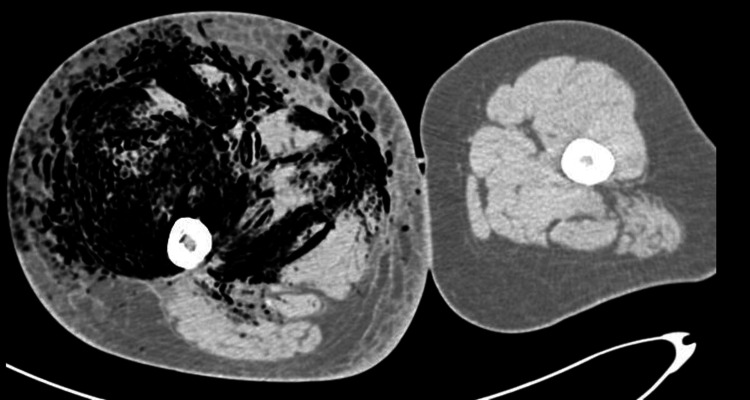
Axial CT scan of the right thigh showing severe soft tissue edema and extensive gas collections dissecting through subcutaneous fat and muscular compartments, consistent with necrotizing fasciitis. There is significant swelling and disruption of normal fascial planes.
